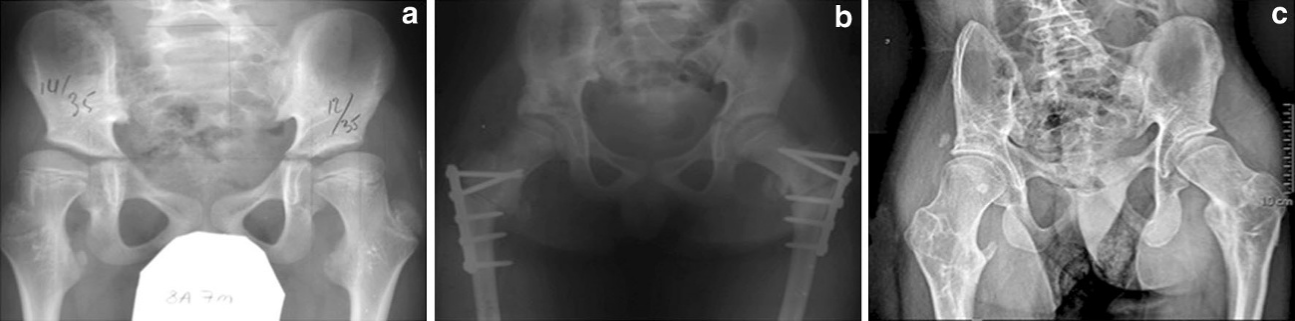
a Pre-operative X-ray of a 8-year-old boy with bilateral hip excentration. b Postoperative X-rays: *right hip*: Dega procedure and femoral shortening, varus and derotation osteotomy. *Left hip* femoral osteotomy alone. c Long-term follow-up
